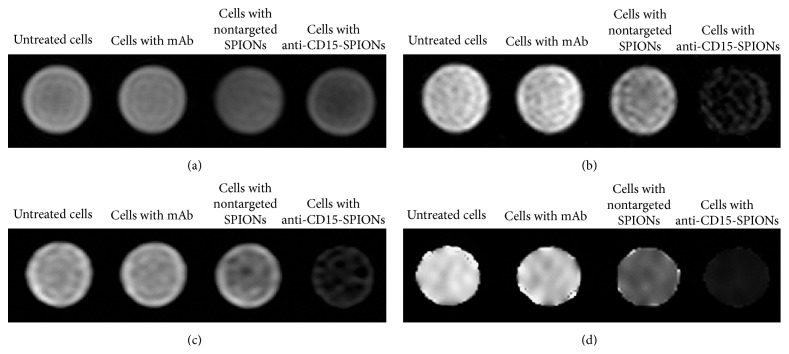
In vitro MRI of cells binding to anti-CD15-SPIONs. Cells incubated with anti-CD15 SPIONs show decreased signal intensity on T1-weighted imaging (a), T2-weighted imaging (b), and T2^*∗*^-weighted imaging (c) and T2 map (d) in comparison with negative control cells.
